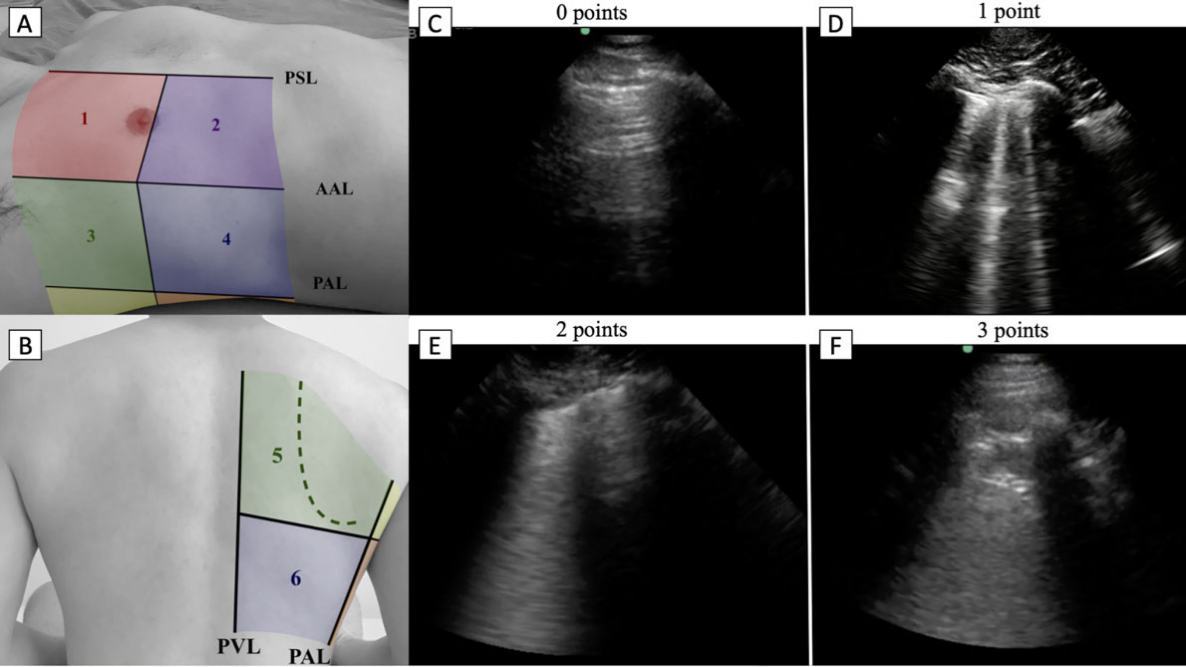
Lung ultrasound zones and scoring. A and B, Zones of lung ultrasound. PSL parasternal line, AAL anterior axillary line, PAL posterior axillary line, PVL paravertebral line. Examples of lung ultrasound findings according to lung ultrasound point. C, Normal aeration of the lung. D, Three well differentiated B-lines, E, Confluent B-line, F, > 1 cm consolidation.
